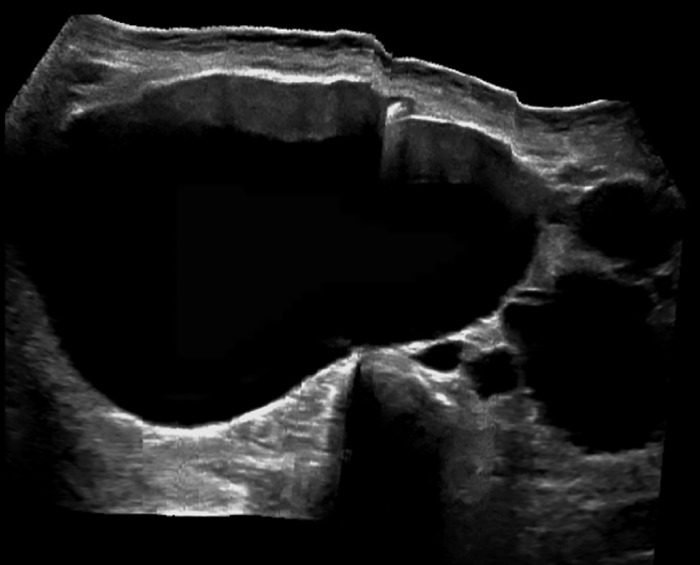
Ultrasonography of right kidney revealing a massive simple renal cyst.
